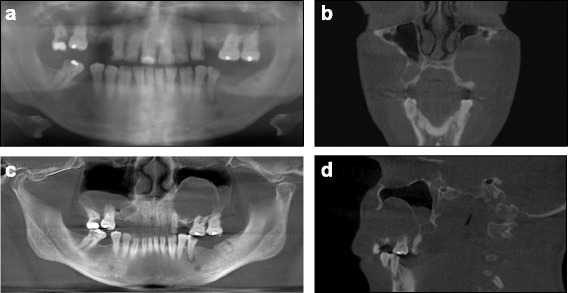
Radiographic findings of patient 26: basal opacity, maxillary bone cyst penetrating into the sinus; a panoramic radiography (Soredex, Cranex); b–d CBCT images (KaVo 3D eXam): coronal plane (b); panoramic reconstruction view (c); CBCT sagittal plane, left sinus (d)
